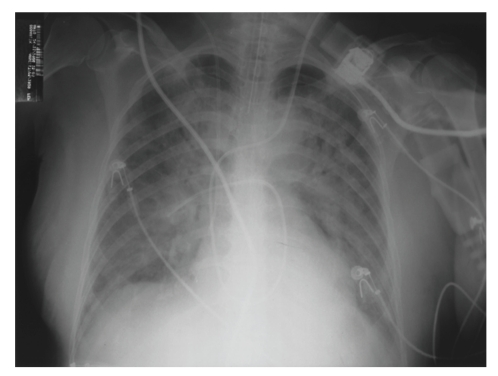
Chest radiograph showing bilateral pulmonary parenchymal infiltrate compatible with ARDS.
